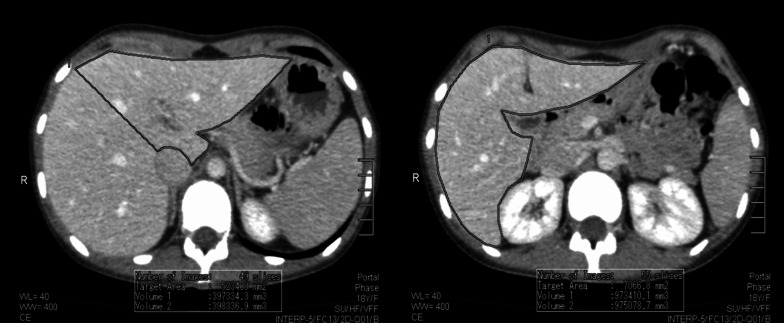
Manual CT volumetry of the liver by Vitrea software
